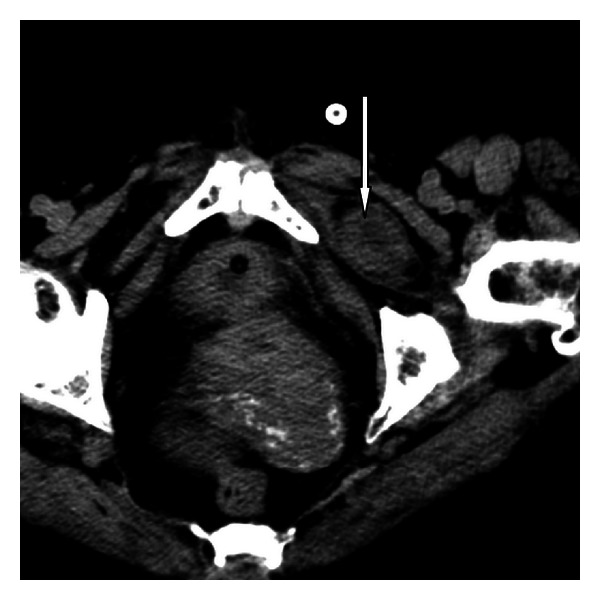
Computed tomography image showing a herniated bowel loop in the obturator foramen on the left.
